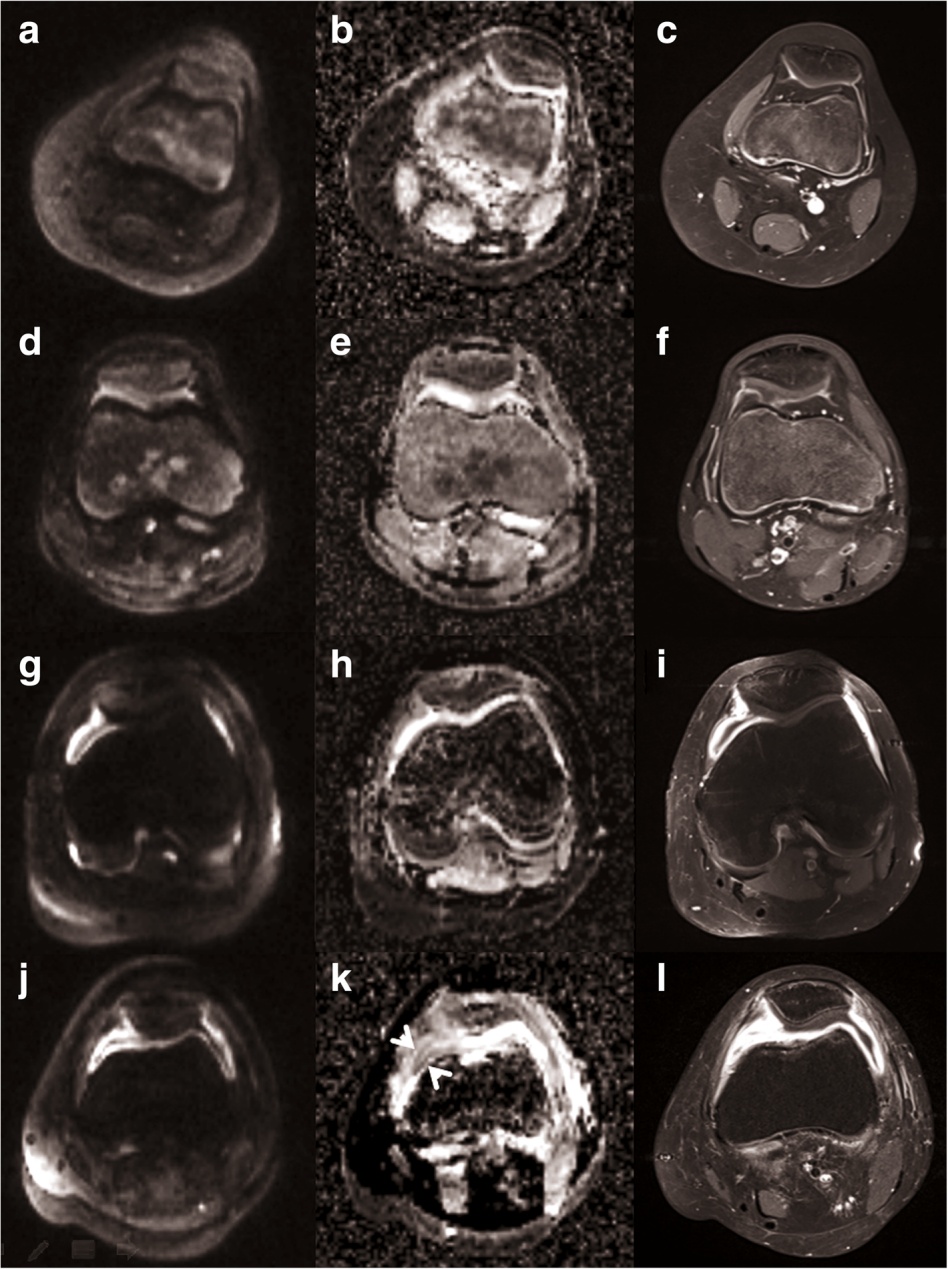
Corresponding transverse cross-section of DWI b = 800 s/mm^2^ (a, d, g, j), ADC map (b, e, h, k), contrast-enhanced T1w imaging (c, f, i, l) in four different patients, rated as not having synovitis (category 1, a-c), showing mild synovial irritation (category 2, d-f), active synovitis with joint effusion (category 3, g-i) and synovitis aggravated by synovial thickening (category 4, j-l) on contrast enhanced MRI as the diagnostic standard. The fourth patient (j-l) was rated as category 3 on DWI, since the synovial layer appears less prominent and since the ADC map nicely delineates synovium from joint effusion (arrow heads) and periarticular oedematous signal increase. Contrast-enhanced MRI may overestimate the extent of synovial proliferation in the presence of perisynovial inflammation and oedema in this patient
